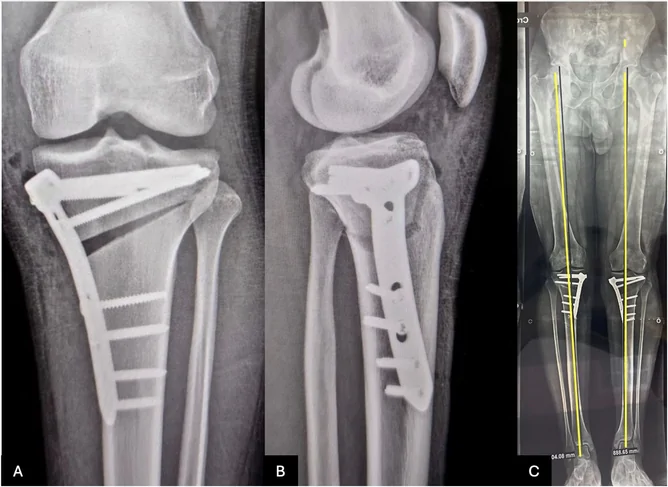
Postoperative radiographs showing medial open‐wedge high tibial osteotomy fixed with a TomoFix plate. (A) Anteroposterior and (B) lateral views of the left knee showing maintained lateral hinge integrity. (C) Full‐length standing anteroposterior radiograph (long‐leg film) of both lower limbs showing restored mechanical axis alignment.
